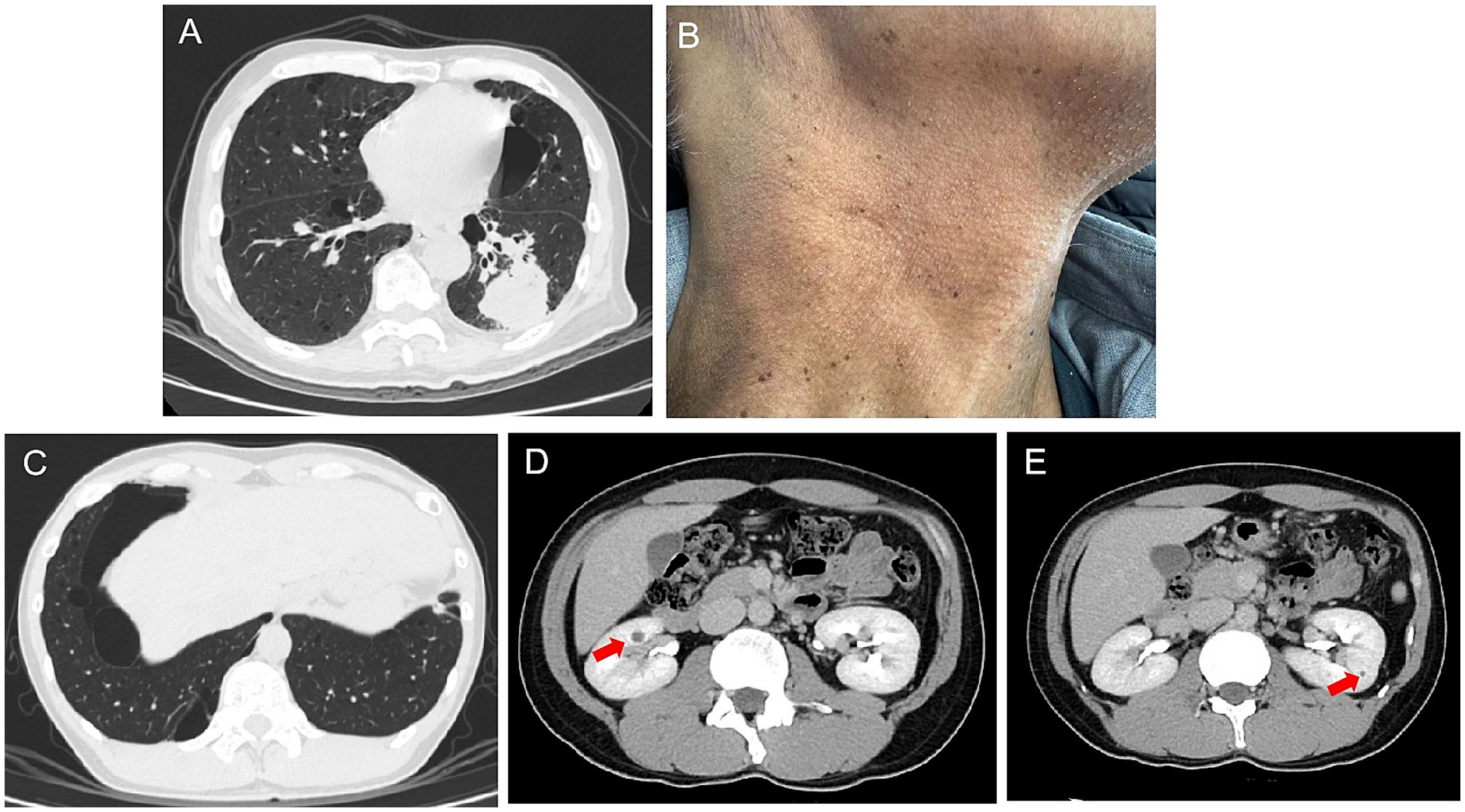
(A) Chest computed tomography (CT) scan of the patient. (B) Cutaneous fibrofolliculomas observed on this patient’s neck. (C) Chest CT scans of his son reveal multiple thin-walled parenchymal cysts. (D,E) Enhanced abdominal CT of his son indicates tumors in both of his kidneys, indicated by a red arrow.
